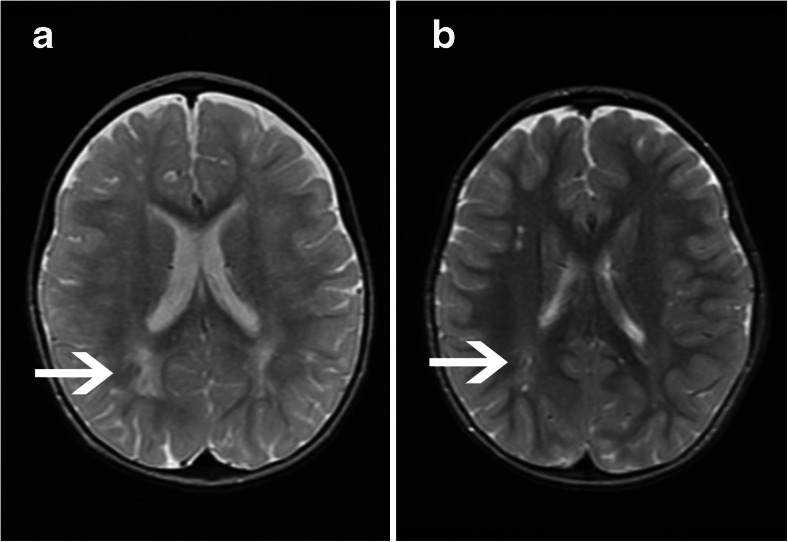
Longitudinal brain magnetic resonance imaging (MRI). (a) The patient was 2 years of age at first MRI. (b) The patient was 4.5 years old at the second MRI. The arrows indicate a delay in myelination in the occipital region (slices are at slightly different levels, due to the quality of MRI). Please note the presence of enlarged Virchow Robin spaces in the white matter in the second MRI (not present in the first MRI)
